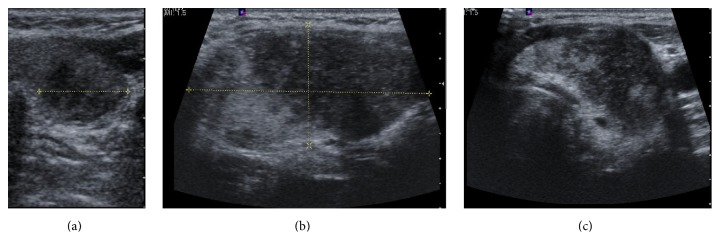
Thyroid ultrasound: (a) left midlobe dominant nodule in transverse view 1.4 × 1.3 × 1.5 cm (total lobe volume 8 mL) before FNA; (b) left lobe nodule in sagittal view 3.9 × 2.3 × 2.2 cm (total lobe volume 15.5 mL) after FNA; (c) left thyroid lobe 1 month after second FNA (total lobe volume 6.5 mL).
